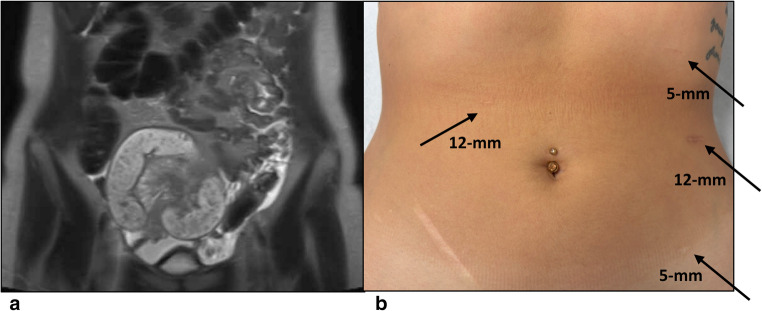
a – MRI with ASBO after open appendectomy (case 2). b – Postoperative cosmetic result with minimal scarring
